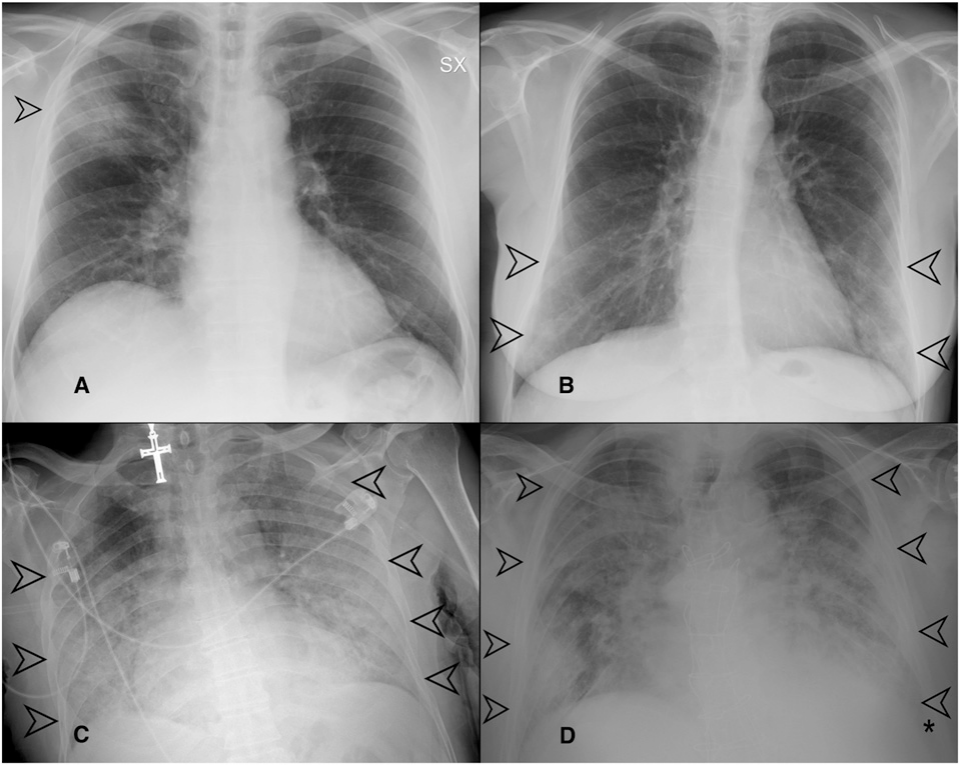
(A–D) Examples of chest X-ray (CXR) score assignment. (A) Chest X-ray showing a focal area of ground-glass opacifications (GGOs) in the upper field of the right lung (black arrow). The involvement of the right lung was < 25%; therefore, the CXR severity score assigned was 1. (B) Chest X-ray showing bilateral areas of GGOs involving the lower lung zones (black arrows). On both the left and right lungs, the involvement was < 50%; therefore, the score was 2 for each lung, with a global score of 4. (C) Chest X-ray showing huge areas of GGOs with bilateral involvement (black arrows), and saving of the upper field of the right lung; the extension on the left side was > 75% (score 4), whereas the involvement on the right side was < 75% (score 3); therefore, the overall score was 4 + 3 = 7. (D) Chest X-ray showing bilateral involvement, with areas of GGOs and consolidation (black arrows) involving all the lung fields. On both the left and right lungs, the involvement was > 75% (score 4); therefore, the global score was 4 + 4 = 8.
